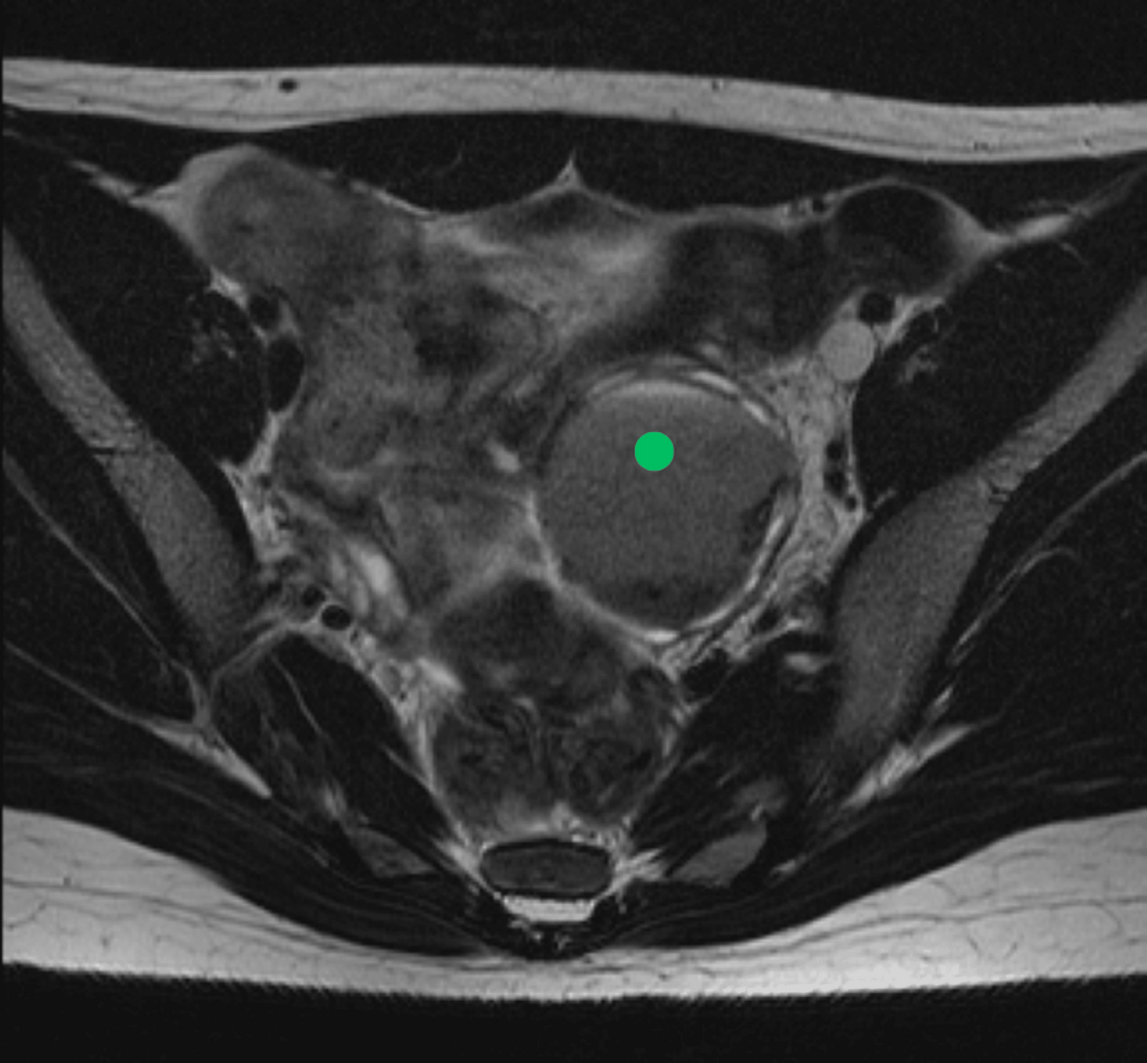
Axial T2 MRI Green dot: left ovarian endometrioma MRI: magnetic resonance imaging
